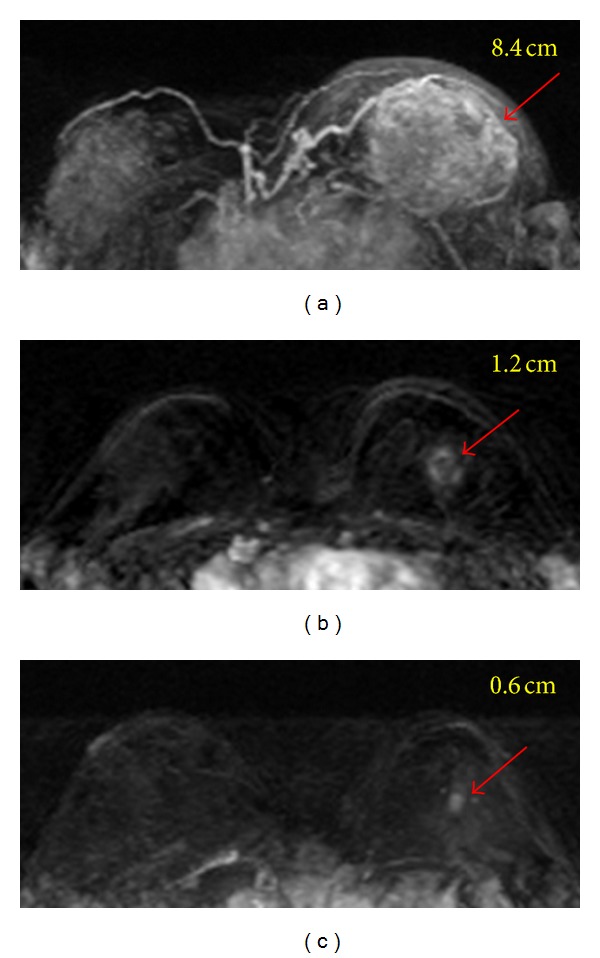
A 35-year-old patient with invasive ductal cancer in the left breast. From (a) to (c), the maximum intensity projection (MIP) images of pretreatment, F/U-1, and F/U-2 MRI are shown. Despite the large tumor, the boundary is clearly visible and this is a mass lesion. The diagnosed tumor size before treatment is 8.4 cm. This cancer is considered as inoperable and is recommended to receive NAC. After 2 cycles of treatment the tumor has shrunk to 1.2 cm, and the size is further decreased to 0.6 cm after completing NAC. Without NAC this patient will need mastectomy and will have a high risk of positive margin. NAC allows this patient to receive breast-conserving surgery, with a good cosmetic outcome.
